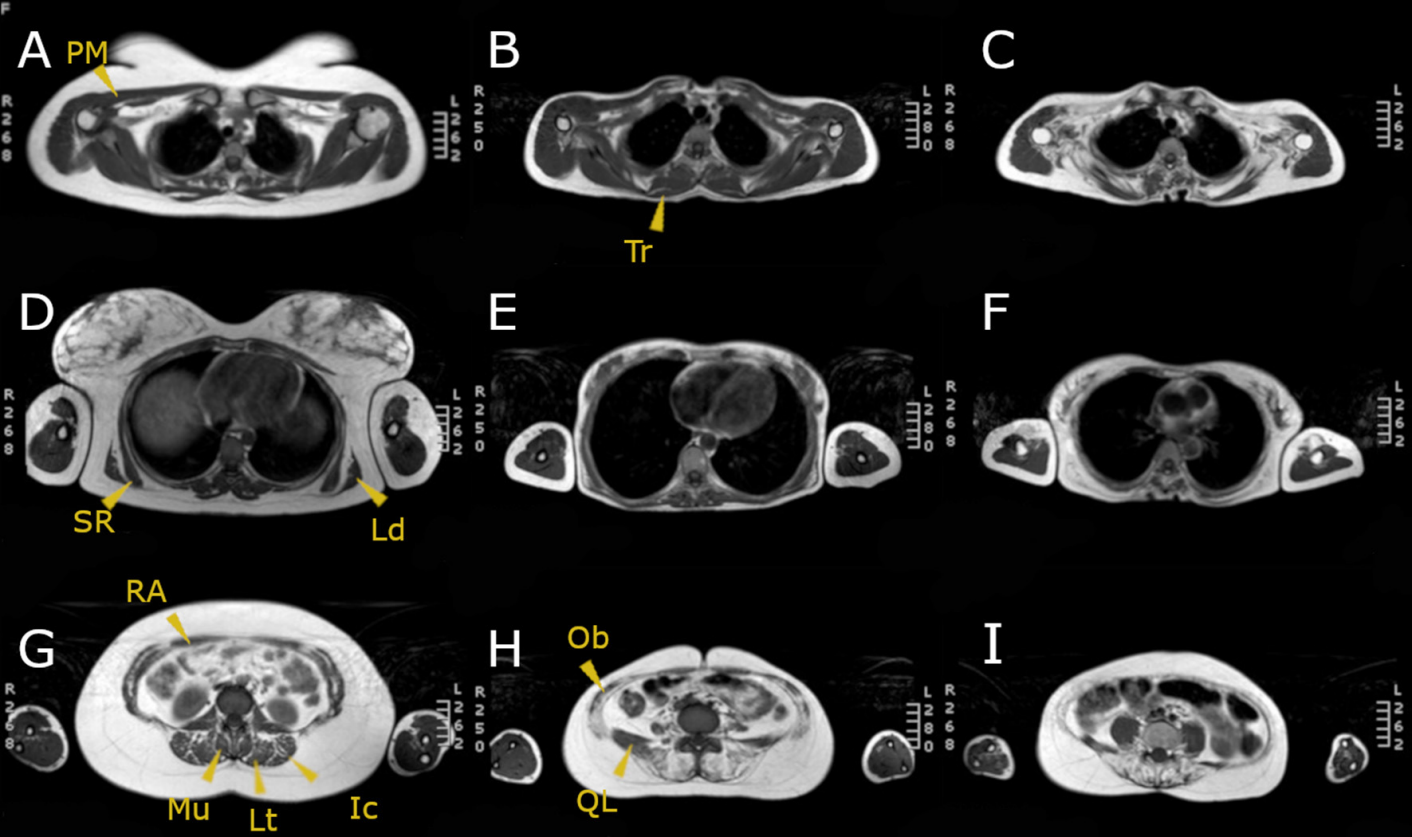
MRI T1w of three LOPD patients: (A,D,G) correspond to a patient with mild involvement; (B,E,H) correspond to a patient with moderate muscular involvement; (C,F,I) correspond to a patient with severe muscular involvement. Yellow arrowheads point the muscles assessed. PM, pectoralis major; SR, serratus; Ld, latissimus dorsi; RA, rectus abdominis; Mu, multifidus; Lt, longissimus thoracis; Ic, iliocostalis; Tr, trapezius; Ob, obliquus externus and internus; QL, quadratus lumborum.
